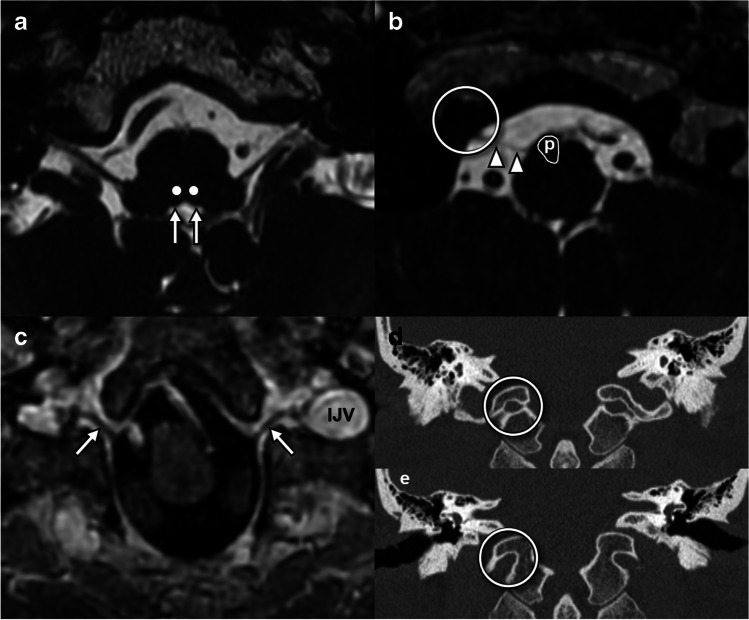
Imaging anatomy of the hypoglossal nuclei and intramedullary fascicles and cisternal and skull base segments. a Axial FIESTA sequence through the upper medulla at the level of the inferior olivary nucleus demonstrates the hypoglossal eminence (arrows) at the floor of the fourth ventricle. The expected location of the hypoglossal nuclei (dots) can be inferred from this landmark. b Axial FIESTA sequence through the lower medulla below the obex demonstrates HN rootlets (arrowheads) exiting lateral to the pyramidal tracts (p) and into the hypoglossal canal (open circle). c Axial post-gadolinium volumetric T1 sequence through the hypoglossal canal demonstrates the canalicular segment of the HN (arrows) as a filling defect surrounded by venous enhancing venous plexus, passing toward the internal jugular vein (IJV) at the upper margin of the carotid sheath. d Coronal CT at the level of the internal (posterior) os of the hypoglossal canal where the canal has a circular shape (circle). e Coronal CT at the level of the external (anterior) os of the hypoglossal canal with a “bird’s beak” appearance (circle)
